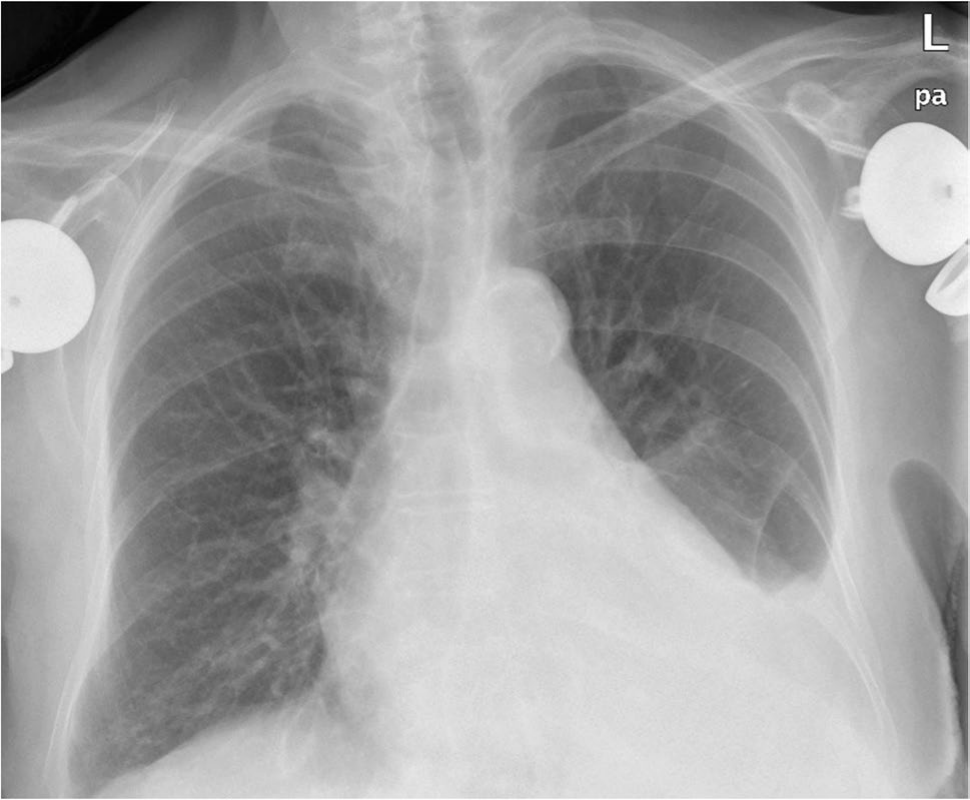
Anteroposterior chest radiograph showing a left-predominant pleural effusion
